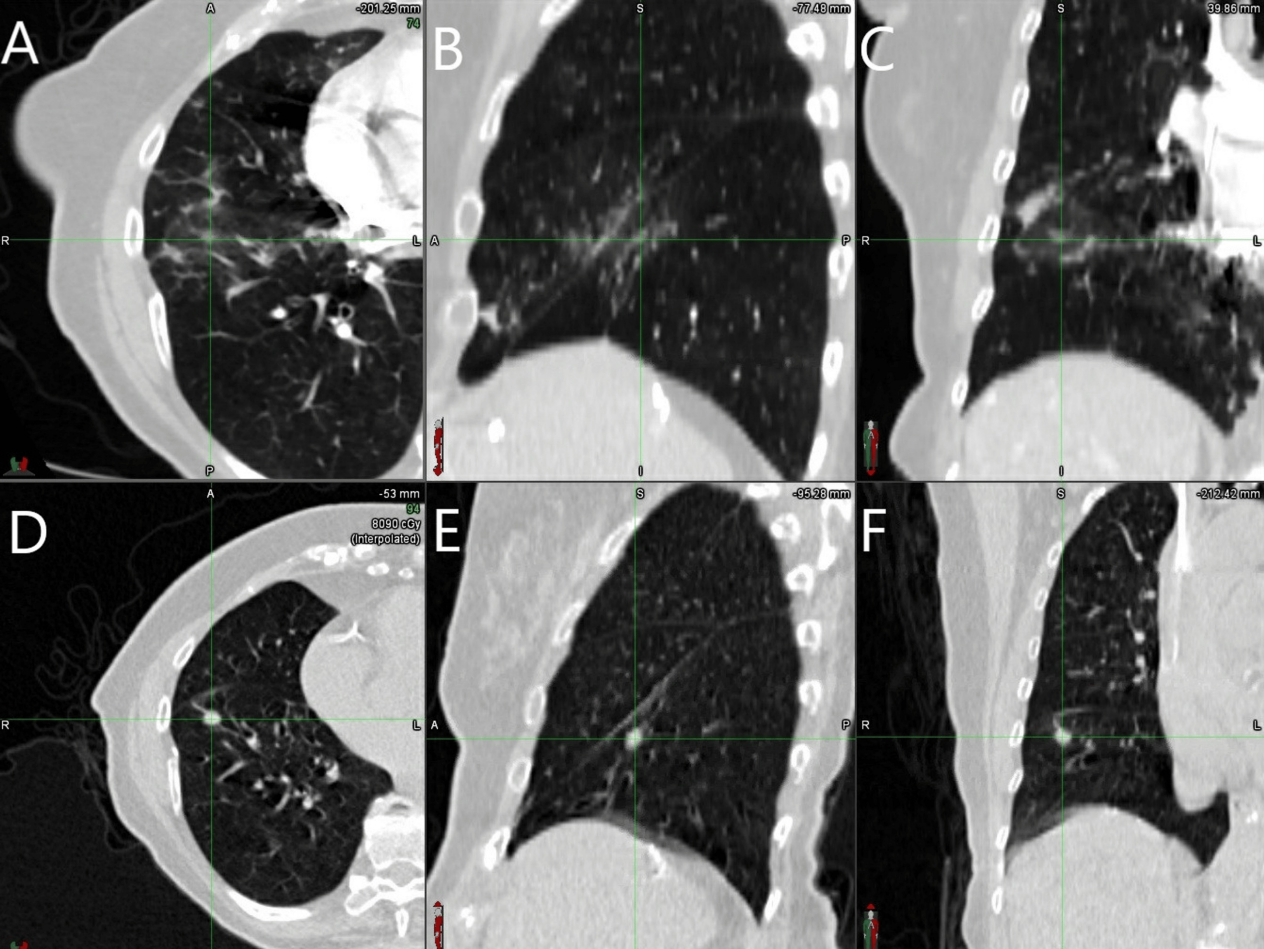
Post-treatment CT images at 20 months (A, B, C) registered with planning CT images (D, E, F), with green crosses indicating the original tumor location on axial (A and D), sagittal (B and E), and coronal (C and F) views CT: computed tomography
